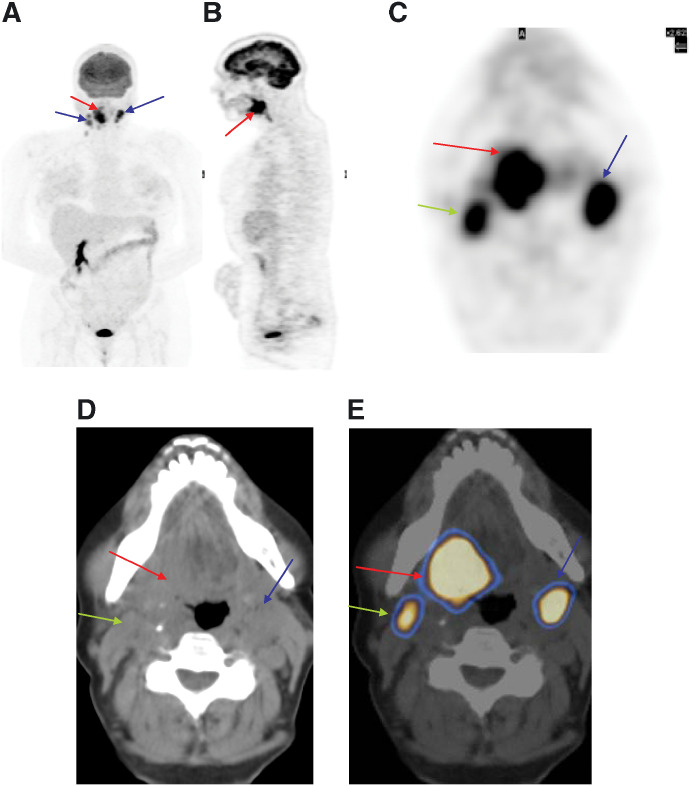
A 42-year-old male with squamous cell carcinoma of tongue. (A) ^18^F-FDG PET/CT multiple intensity projection image shows the primary site (red arrow) with bilateral cervical nodes (blue arrows). (B) Sagittal and (C) transaxial images show abnormal uptake in the known primary (posterior part of the tongue) and both cervical regions. (D) CT transaxial section reveals a lesion in the base of the tongue along with left cervical node enlargement. (E) Fused ^18^F-FDG PET/CT transaxial section at the same level reveals the exact anatomical site of ^18^F-FDG uptake in the right side of the tongue base extending across the midline and level II left cervical lymph node. The ^18^F-FDG activity in the right cervical region correlates to the right sternocleidomastoid muscle (green arrow) (a normal variant).
